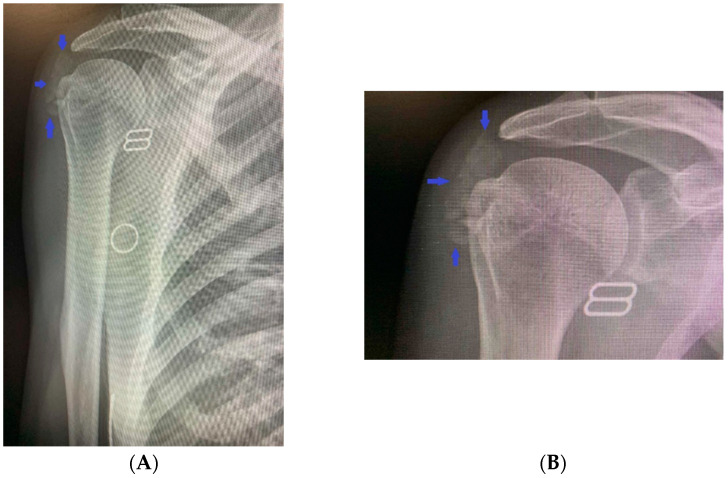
(A,B) An X-ray of the right shoulder joint revealing calcium deposits in the surrounding soft tissues.
